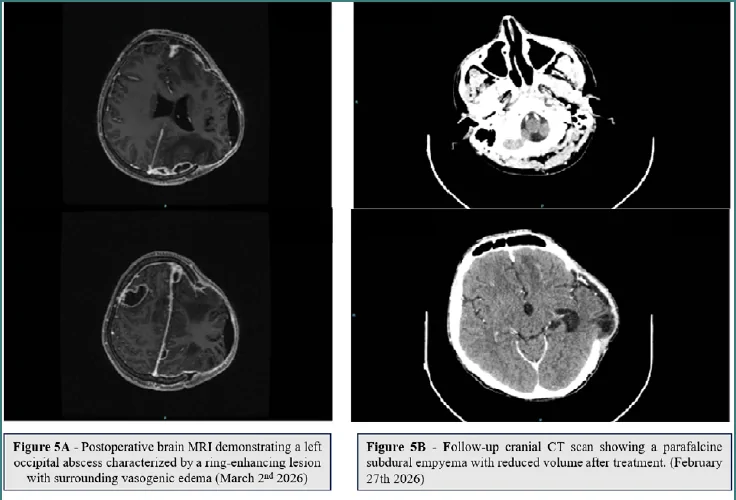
Postoperative neuroimaging demonstrating the evolution of intracranial infection following surgical treatment. A, Postoperative MRI of the brain demonstrating a ring-enhancing left occipital abscess with surrounding vasogenic edema (March 2, 2026). B, Follow-up cranial CT demonstrating a reduced parafalcine subdural empyema after treatment (February 27, 2026).
